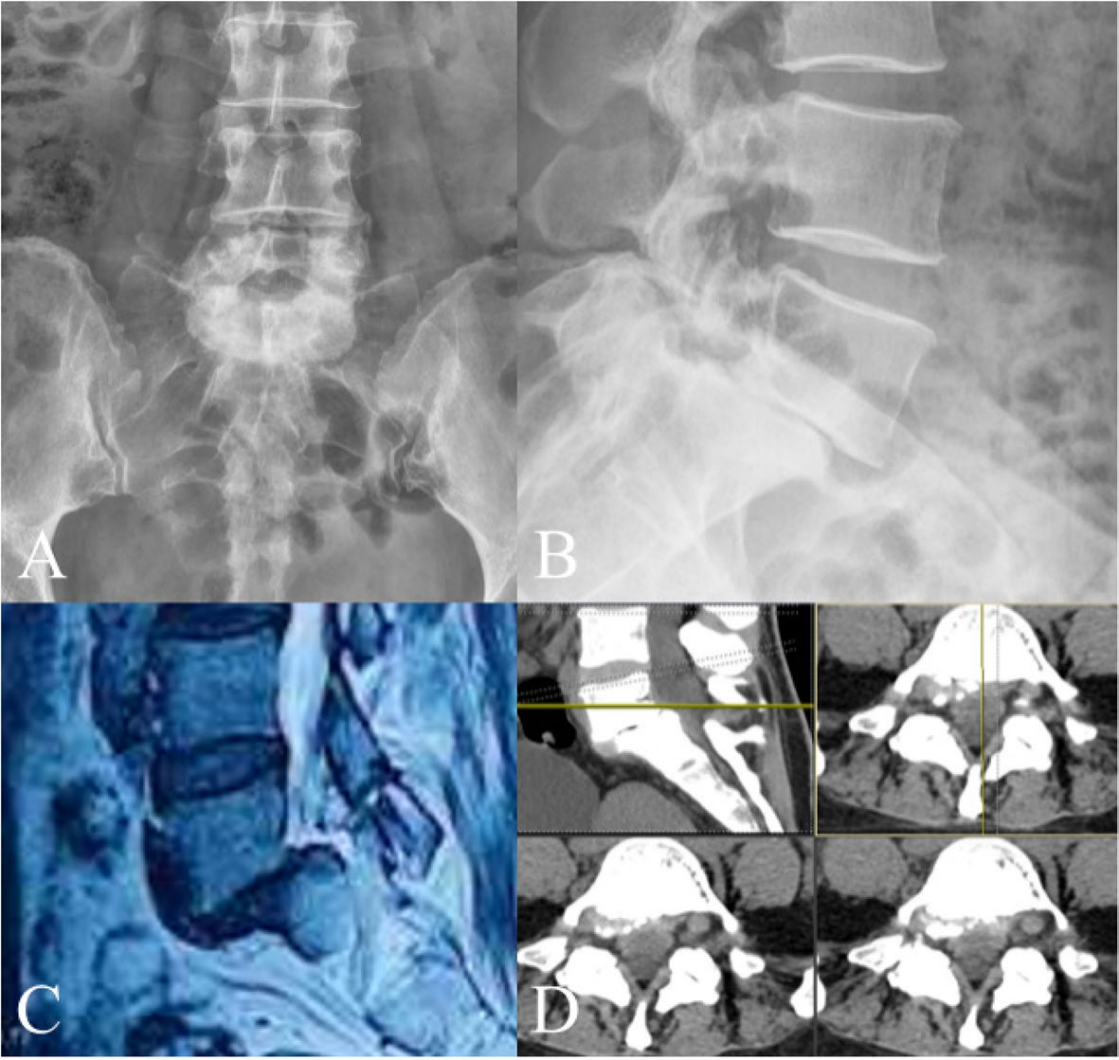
Representative case of a patient before Endo-TLIF. A and B: the fluoroscopic AP (a) and lateral (b) views. C and D: CT scan and MRI of the case with lumbar spondylolisthesis
